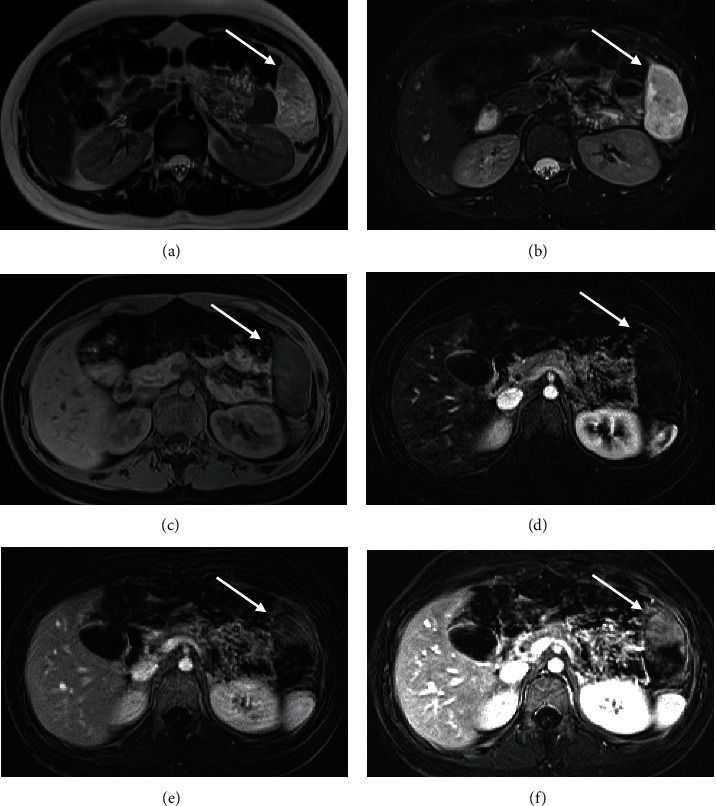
Axial T2-weighted image showing a left upper quadrant lesion with heterogenous hyperintense signal (arrow in (a)). Corresponding axial T2-weighted with fat saturation which shows no evidence of fat signal suppression within the lesion (arrow in (b)). Corresponding T1-weighted image with fat saturation showing no signal hyperintensity excluding the presence of methemoglobin (arrow in (c)). Dynamic contrast-enhanced sequences with subtraction technique (d–f) showed faint early arterial enhancement (arrow in (d)) which progressed in the venous phase (arrow in (e)) and continued to increase in signal intensity during equilibrium phase (arrow in (f)).
